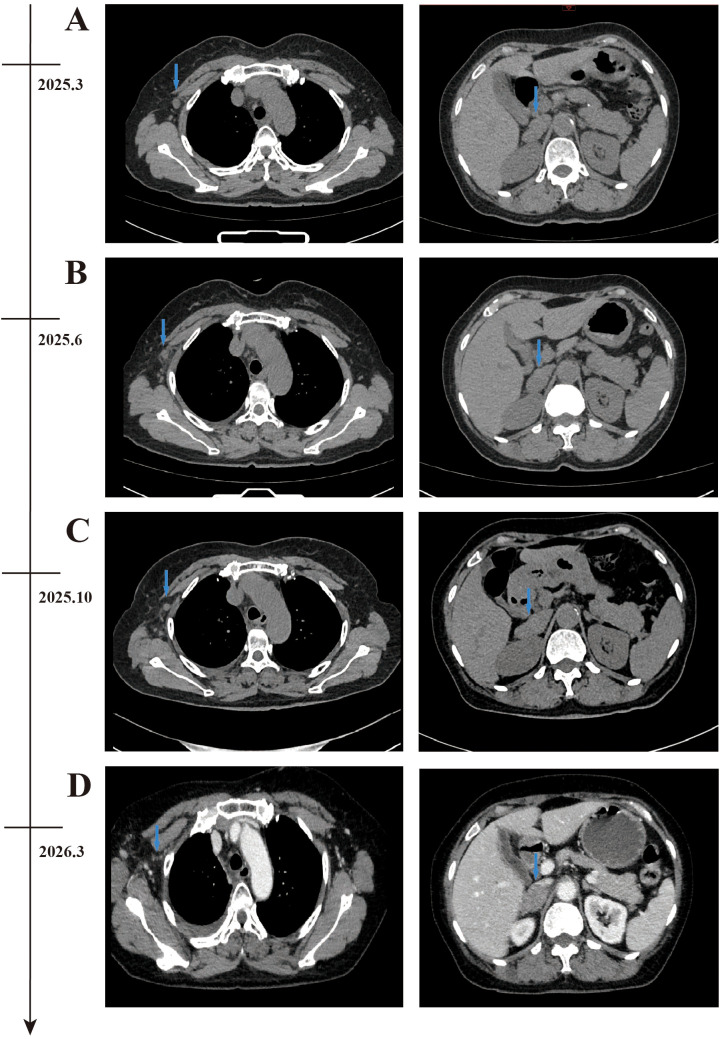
(A) Post-treatment CT (icotinib + radiotherapy): right axillary and adrenal metastases. (B) Post-treatment CT (icotinib + radiotherapy, 3 months): right axillary and adrenal metastases. (C) Post-treatment CT (afatinib, 3 months): right axillary and adrenal metastases. (D) Post-treatment CT (pemetrexed + carboplatin + ivonescimab, 3 months): right axillary and adrenal metastases.
